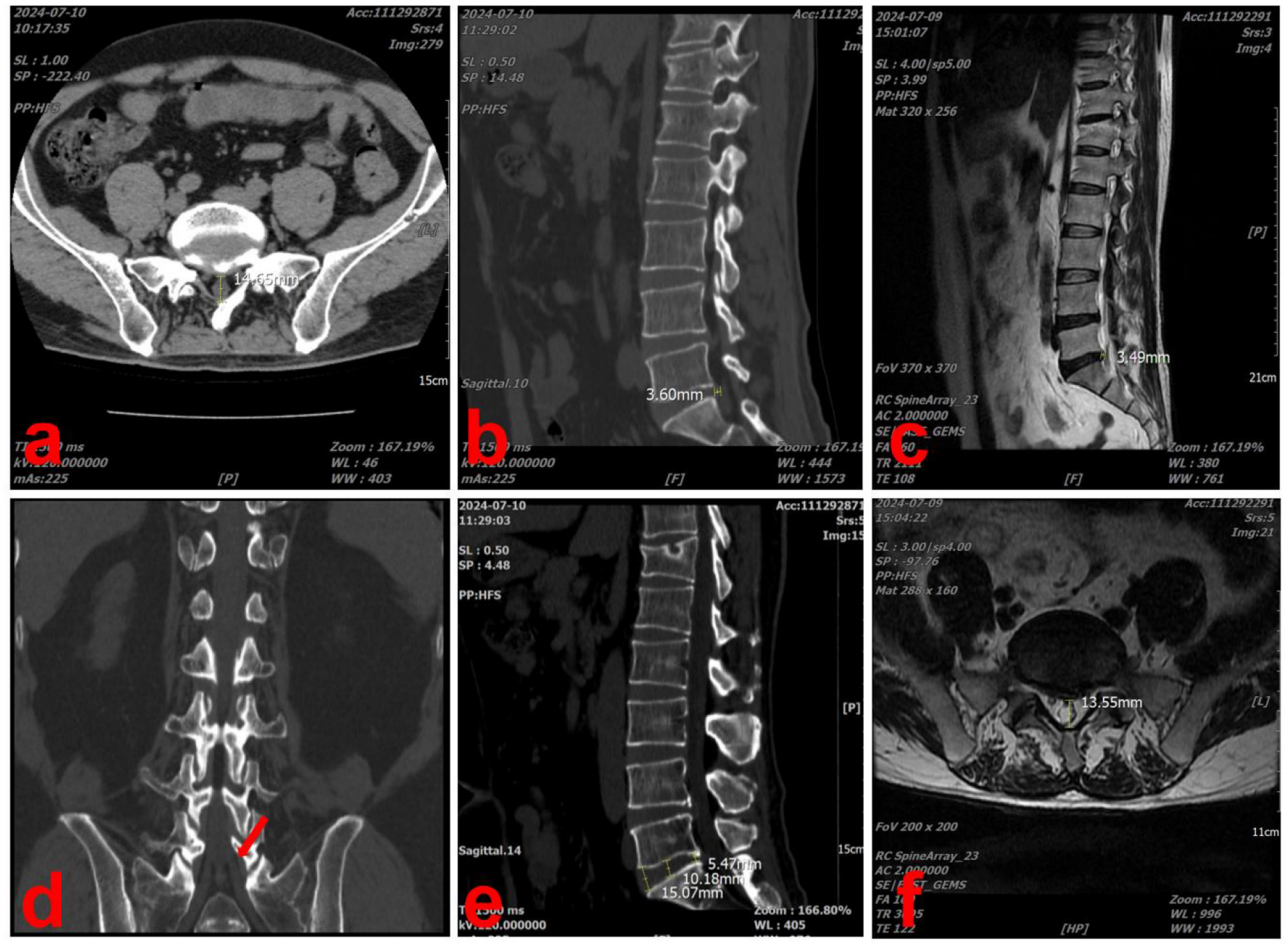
Lumbar spine CT and MRI scans performed at the last visit (2024-07-09). (a) In the CT cross-section, the diameter of the spinal canal at the L5/S1 level was 14.65 mm. (b) In the CT sagittal-section, the herniated disc measured 3.60 mm in length. (c) In the MRI sagittal-section, the herniated disc measured 3.49 mm in length. (d) In the coronal section, there was no longer compression of the S1 nerve root by the herniated disc (red arrow). (e) In the CT sagittal-section, the height of the lumbar intervertebral space was 10.27 mm. (f) In the MRI cross-section, the diameter of the spinal canal at the L5/S1 level was 13.55 mm.
